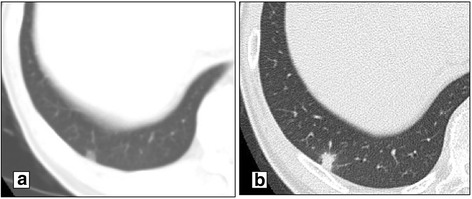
Imaging studies of the second pulmonary metastasis. Chest CT (at the same time when another nodule was found in the lower lobe of the left lung) shows a tiny nodule in the lower lobe of the right lung. It is difficult to determine whether this nodule is a metastatic tumor or an inflammatory nodule. a Follow-up chest CT (94 months after curative resection of the primary pancreatic tumor) shows that this nodule has increased gradually. b It is diagnosed as a pulmonary metastasis from PDAC
